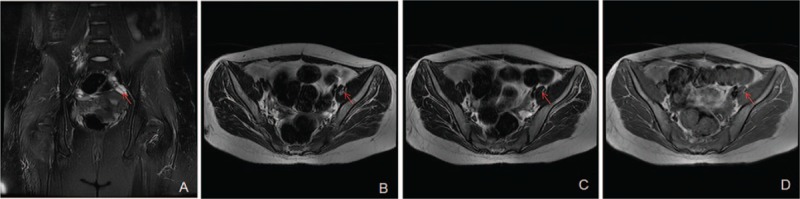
In patient 1, MRI scanning detected a patchy lesion with short T1 and long T2 signal change on the posterior side of the left ovary (red arrow); the fat suppression image showed a low signal with long T1 and short T2 in the center. The size of the lesion was approximately 0.6 × 0.9 cm^2^, and the T2-weighted fat suppression image showed a local signal reduction. After teratoma excision, the right ovary had a clear image of follicles, and no abnormal signal was detected.
